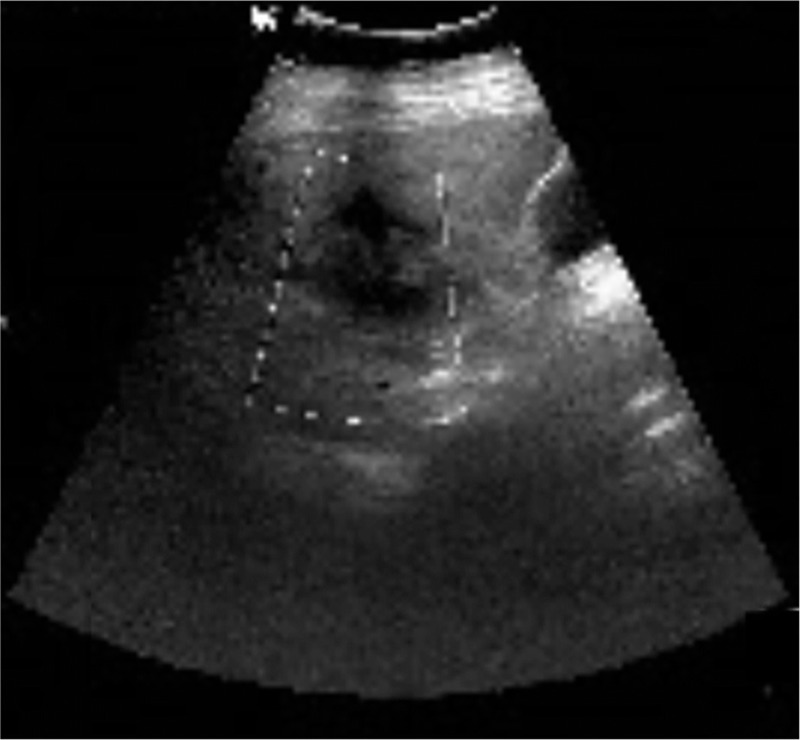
Ultrasound image of the liver showed uneven fatty liver and an inhomogeneous echo zone in the surgical area of the liver, with no blood flow signal.
